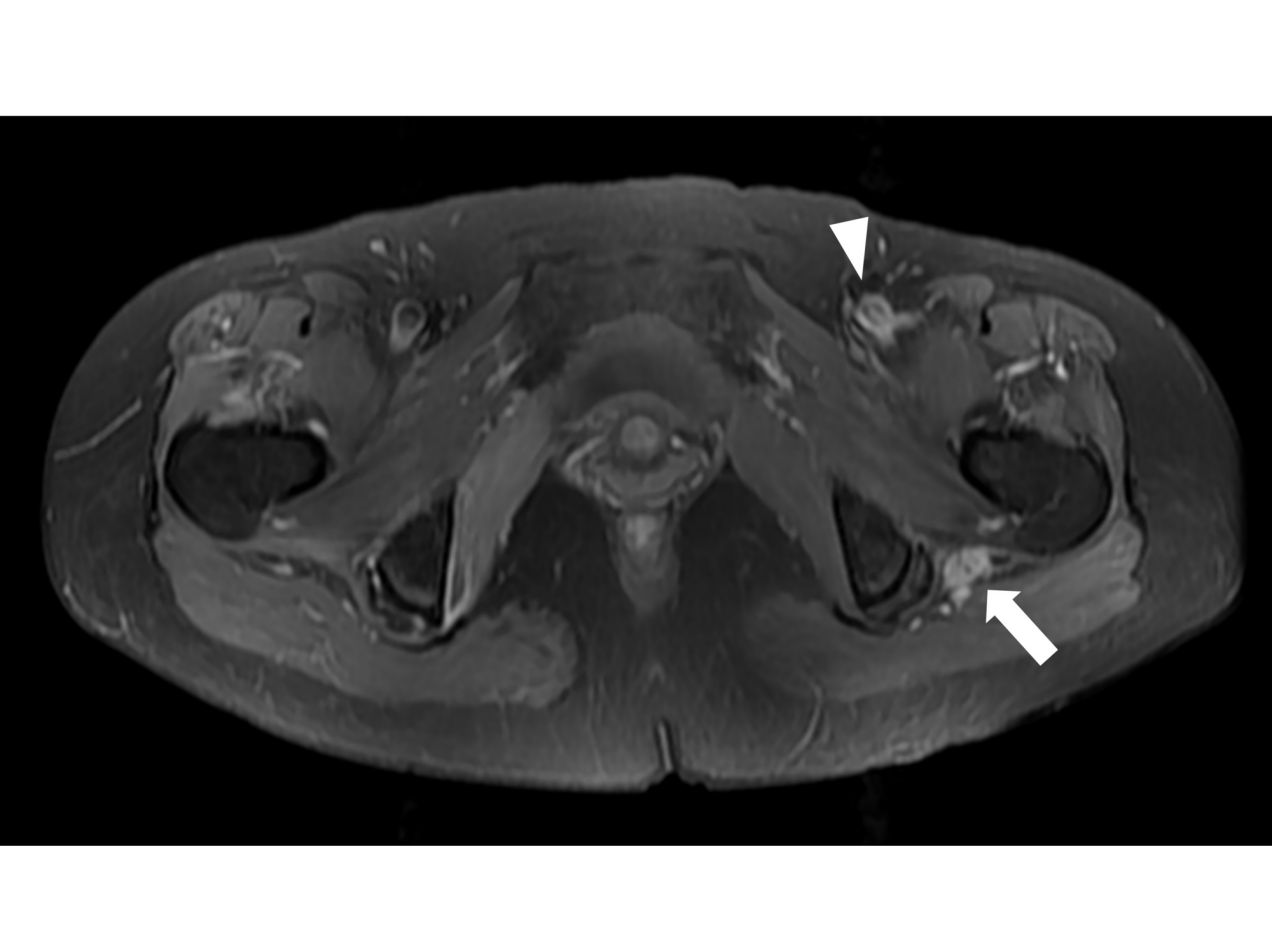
Initial magnetic resonance image (MRI) finding. In T2-weighted axial image after contrast enhancement,  asymmetrically diffuse swelling with increased signal intensity and contrast enhancement of the left sciatic nerve (arrow) was observed. Also mild swelling with T2-hyperintensity of the left femoral nerve (arrowhead) was found in the same plane.
